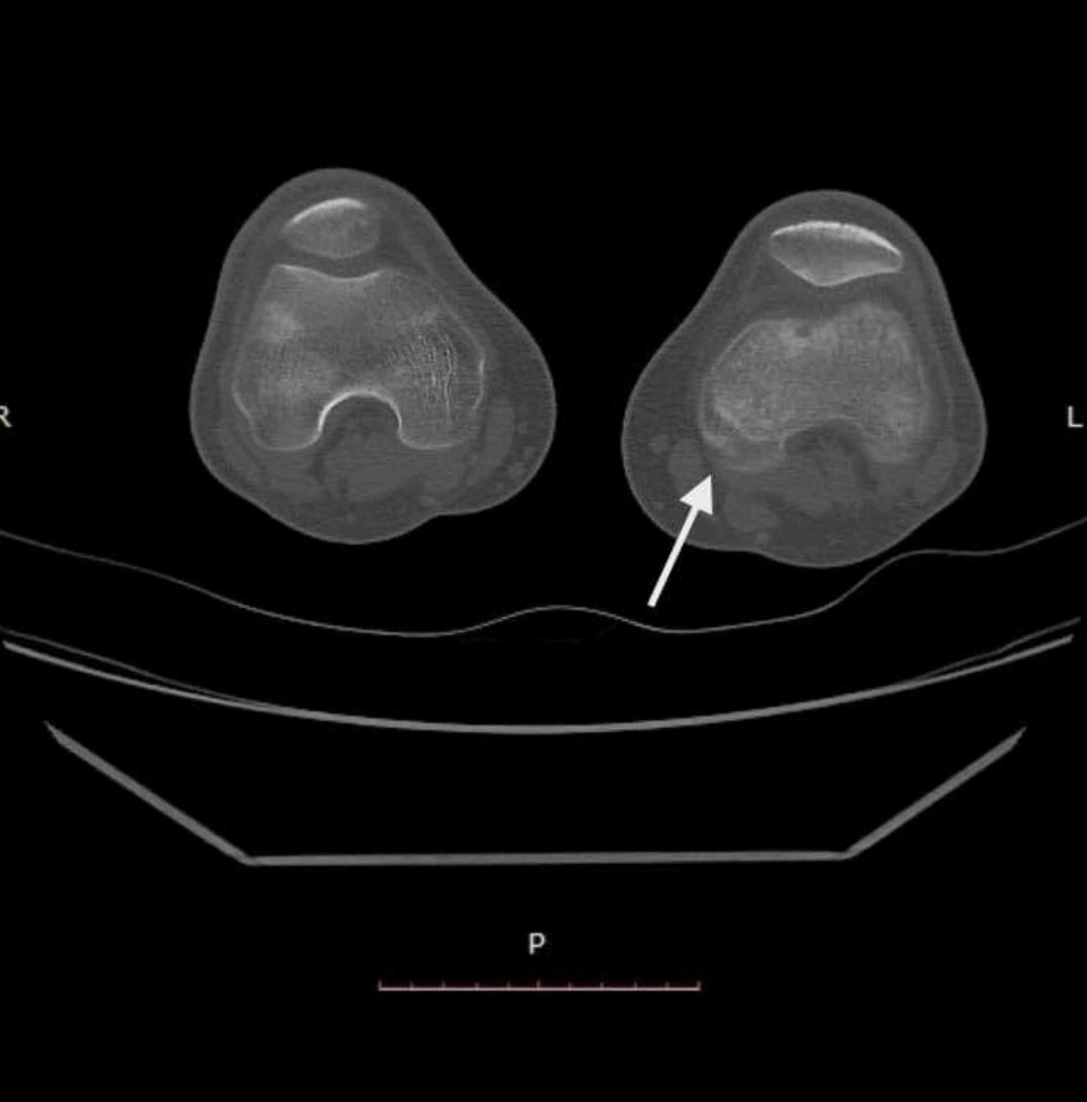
CT scan of the left knee Osteolytic changes in the metaphysis and epiphysis of the left femur (white arrow)
